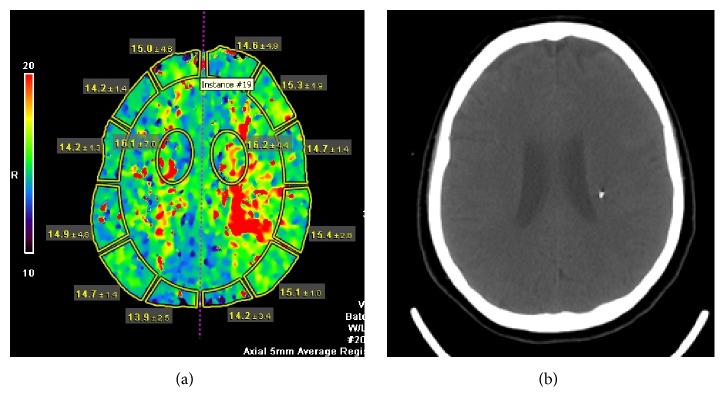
(a) prolongation of the TTP signal in the left central Rolandic white matter. (b) A plain CT scan showing the tip of the BPM in the area of the prolongation of the TTP.
